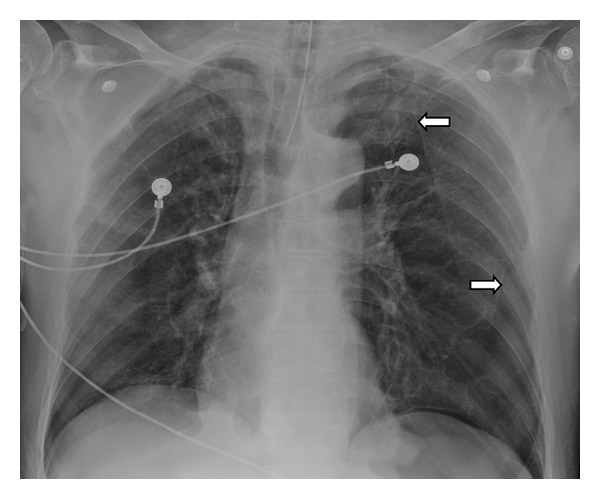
Anteroposterior chest X-ray following endotracheal tube placement showing hyperinflated lungs with multiple areas of hyperlucency on the left lung base and left apex, surrounded by a thin linear demarcation (arrows).
